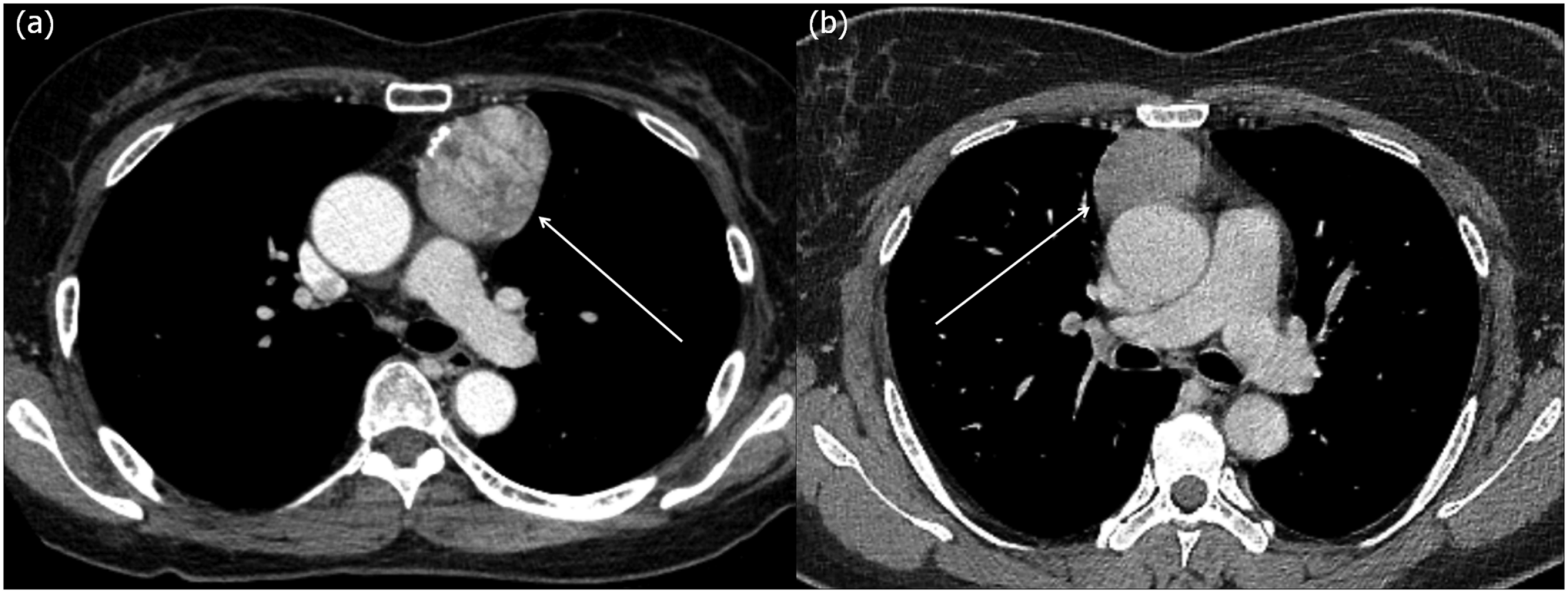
CT images of encapsulated and invasive thymomas. (a) A 61 year old female who underwent surgical resection of an encapsulated thymoma (arrow) (discrete compactness, 0.925; sphericity, 0.703). (b) A 40 year old female who underwent surgical resection of an invasive thymoma (arrow) (discrete compactness, 0.722; sphericity, 0.646). Note that although these two kinds of thymomas cannot be easily differentiated grossly owing to similar CT features, there is a distinct difference in 3D shape features, particularly in discrete compactness, between the encapsulated thymoma and invasive thymoma.
